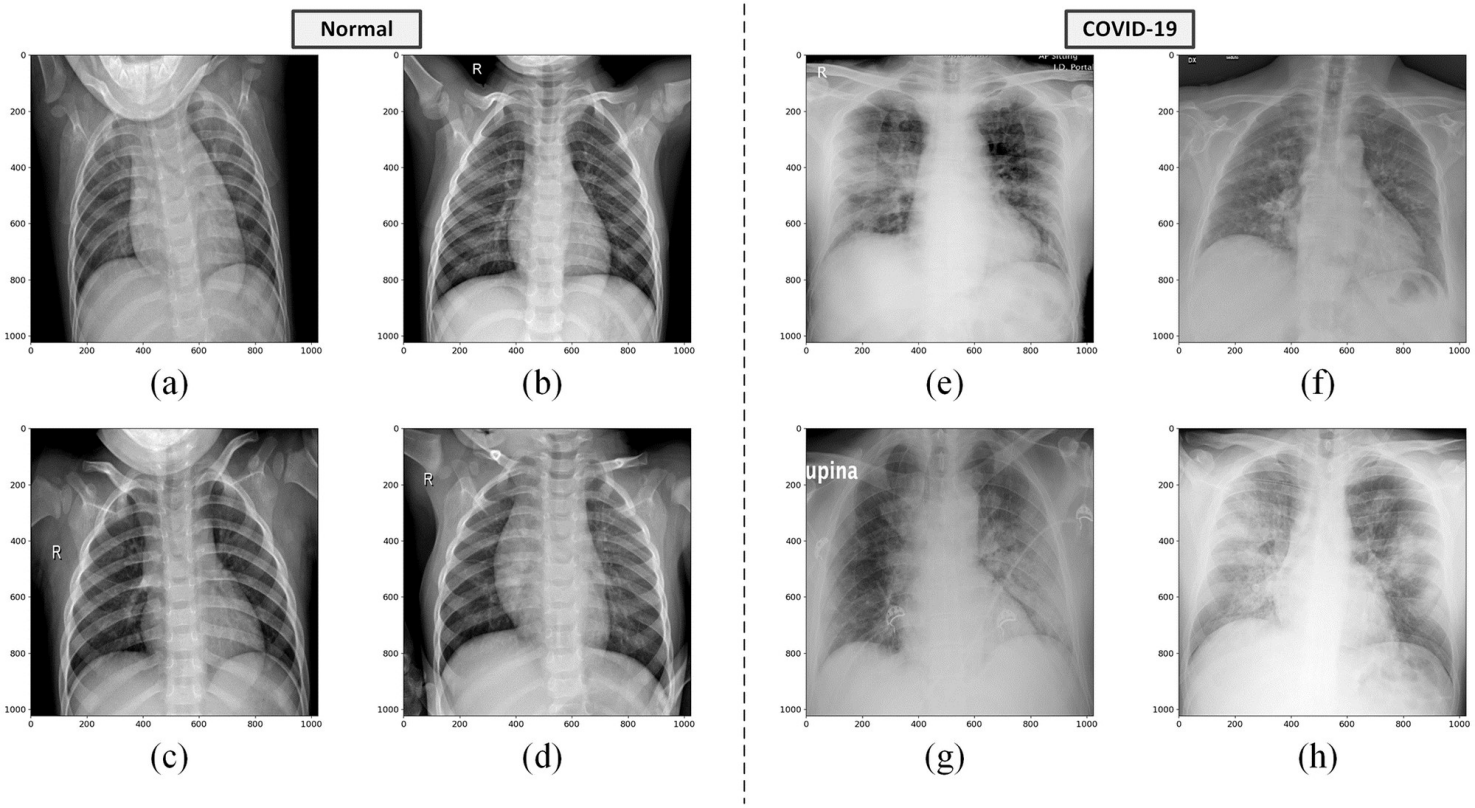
Chest X-ray images of healthy and infected COVID-19 lungs. (a-d) Healthy chest X-ray images. (e-h) COVID-19 chest X-ray images. The images are taken from the COVID-19 Radiography Database [37].
